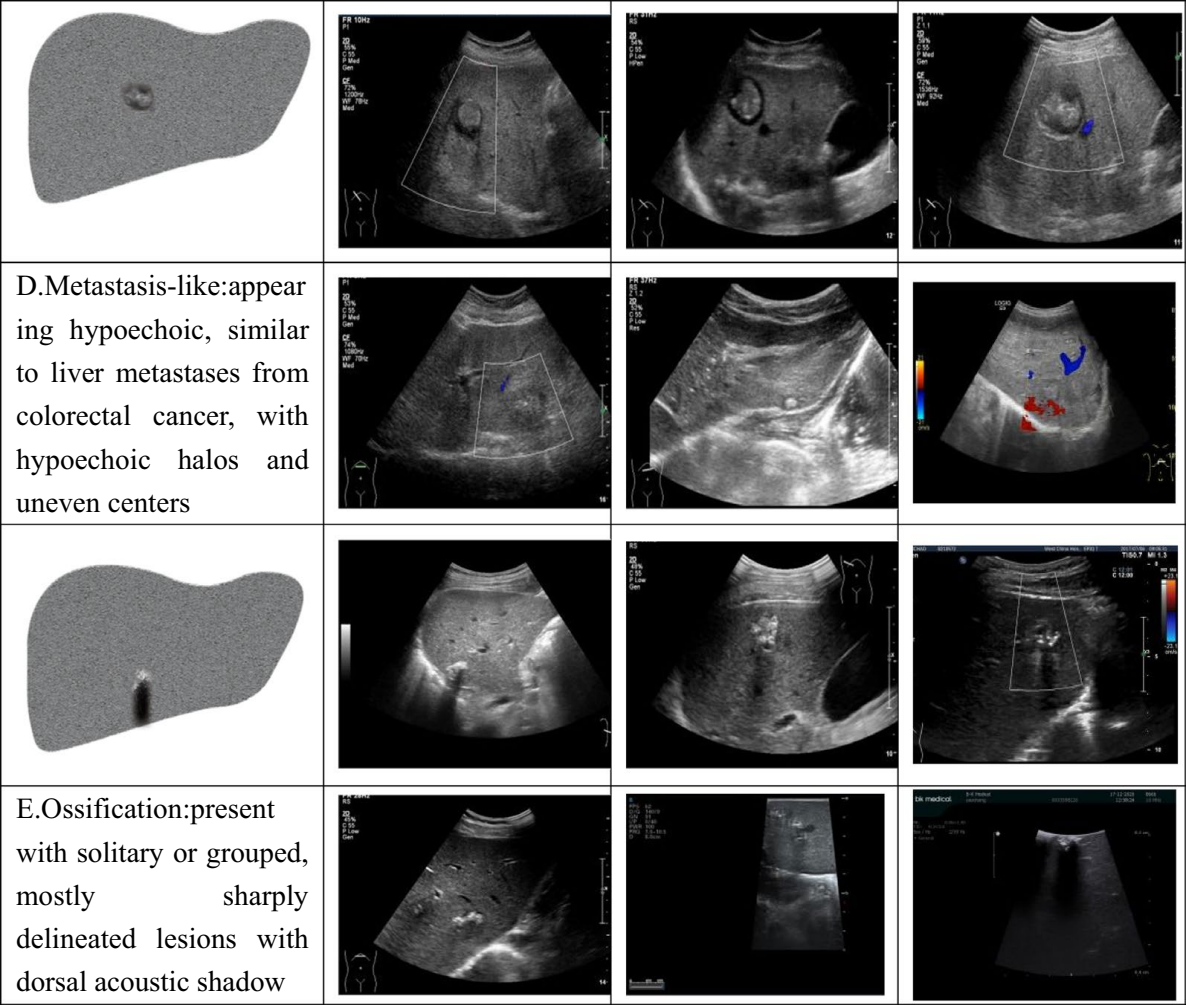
Ultrasound patterns of hepatic alveolar echinococcosis categorized using the *Echinococcus multilocularis* Ulm Classification-Ultrasound (EMUC-US) system. A Hailstorm: irregular shape, indistinct margins, heterogeneous internal echoes with hyperechoic foci and posterior shadowing; B Hemangioma-like: well-demarcated lesion with high or slightly high echogenicity and uniform or heterogeneous internal echoes; C Pseudocystic: hyperechoic rim, unclear borders, peripheral echo heterogeneity, thick cyst wall (> 10 mm), and hypoechoic or anechoic center; D Metastasis-like: hypoechoic lesion resembling colorectal liver metastases, often with a hypoechoic halo and heterogeneous central echotexture; E Ossification: solitary or clustered lesions with sharp margins and prominent dorsal acoustic shadowing due to calcification or ossification. Representative ultrasound images are provided for each subtype
